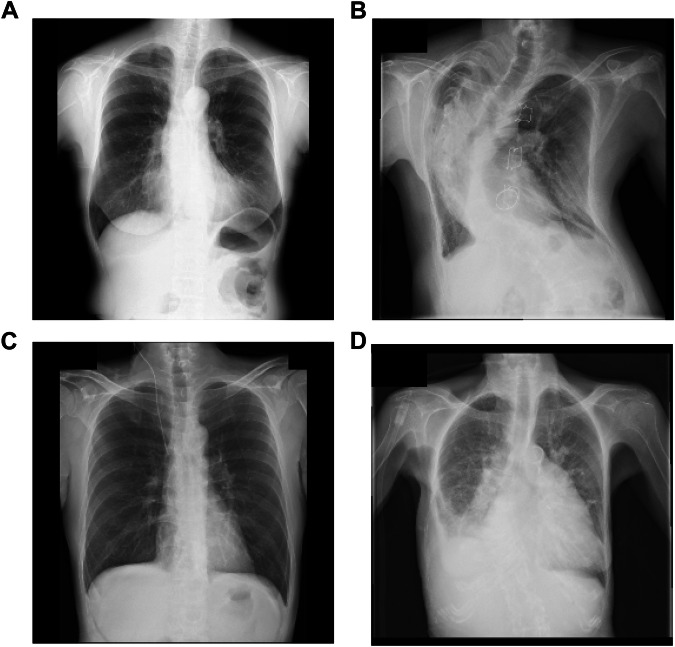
Representative examples of misclassifications by Xp-Bodypart-Checker and CXp-Projection-Rotation-Checker. A Chest radiograph of a female in her 50 s that was misclassified as “Abdomen” by Xp-Bodypart-Checker. B PA chest radiograph of a female in her 40 s that was misclassified as “Lateral” by CXp-Projection-Rotation-Checker. C AP chest radiograph of a male in his 50 s that was misclassified as “PA” by CXp-Projection-Rotation-Checker. D PA chest radiograph of a female in her 80 s that was misclassified as “AP” by CXp-Projection-Rotation-Checker. AP, anterior-posterior; PA, posterior-anterior; AUC, area under the receiver operating characteristic curve
